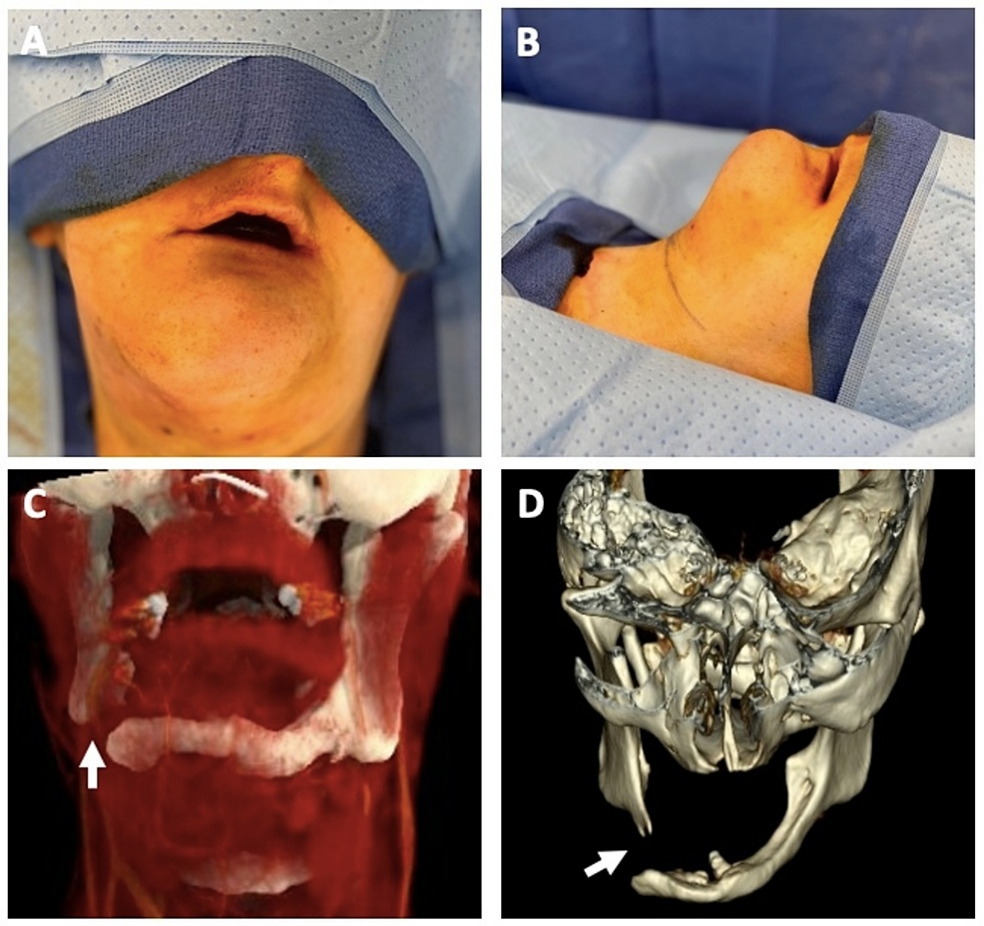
Preoperative mandibular non-union defect Preoperative mandibular non-union defect in frontal view (A), left lateral view (B), CT 3-dimensional reconstruction with soft tissue superimposition in frontal view (C), CT 3-dimensional reconstruction from superior view showing anterior displacement of the left mandible segment. White arrows indicate non-union defect.
